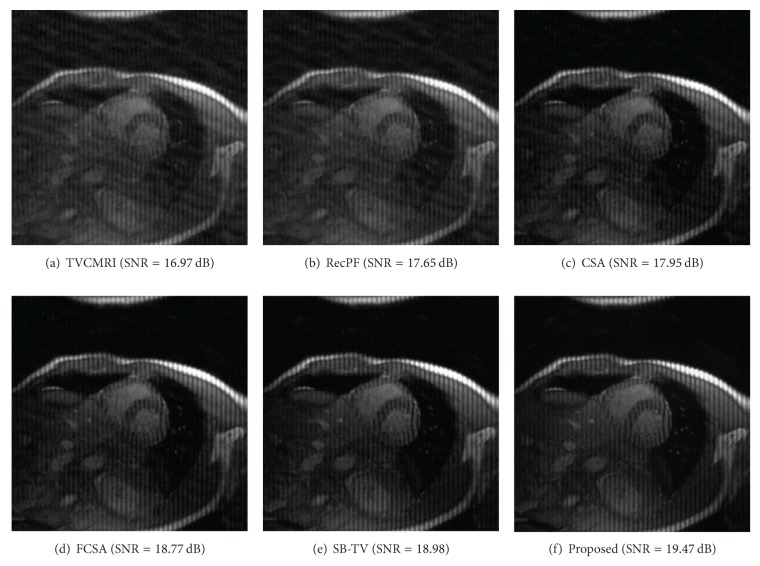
Cardiac MR image reconstruction from 20% samples (Gaussian noise case).
